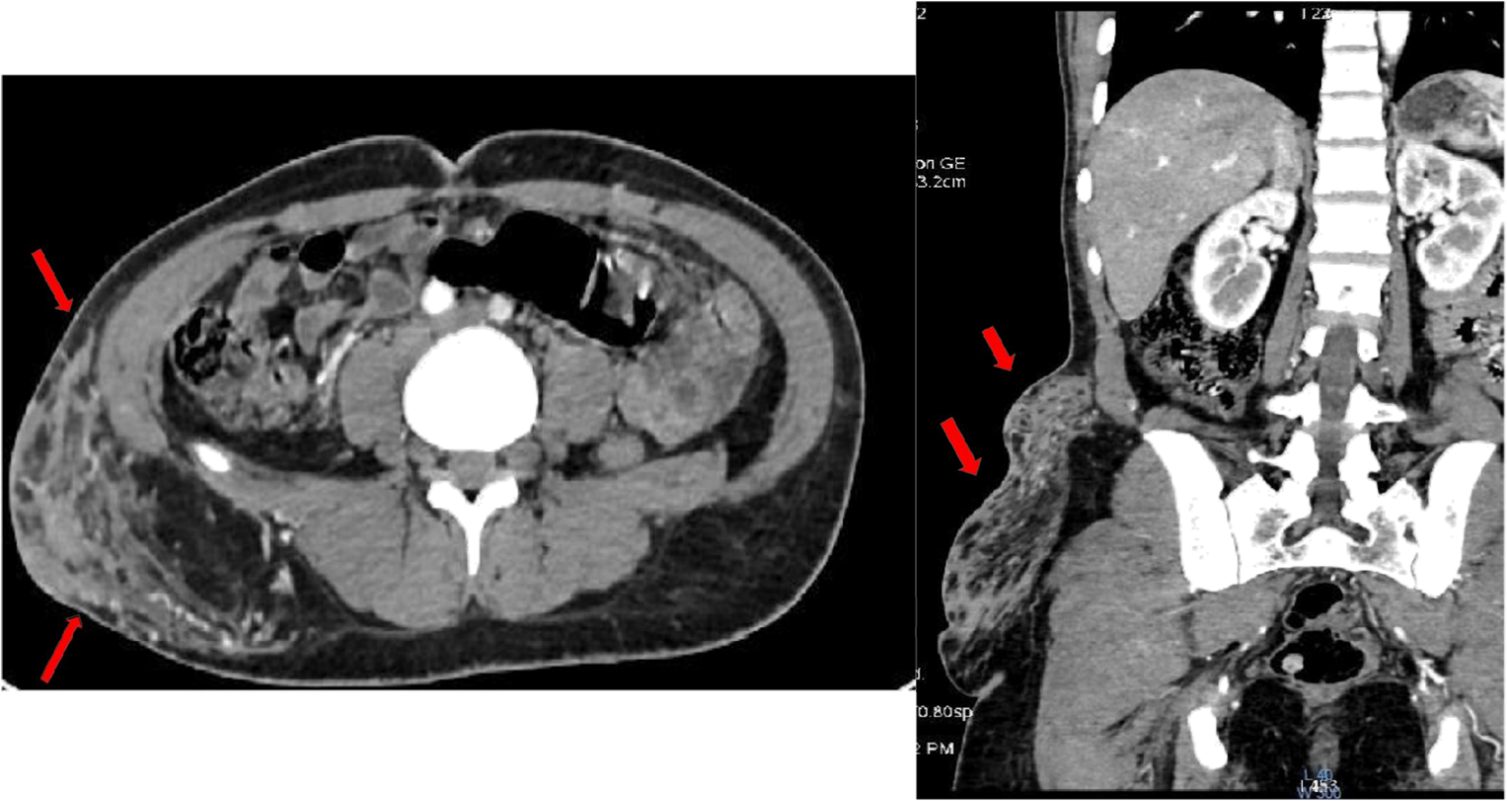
A 31-year-old female with a history of NF1 presenting a subcutaneous mass that feels like a “bag of worms”. CT scan showed an expansive heterogeneous image (arrows) located in the right lumbosacral external region (plexiform neurofibromas)
